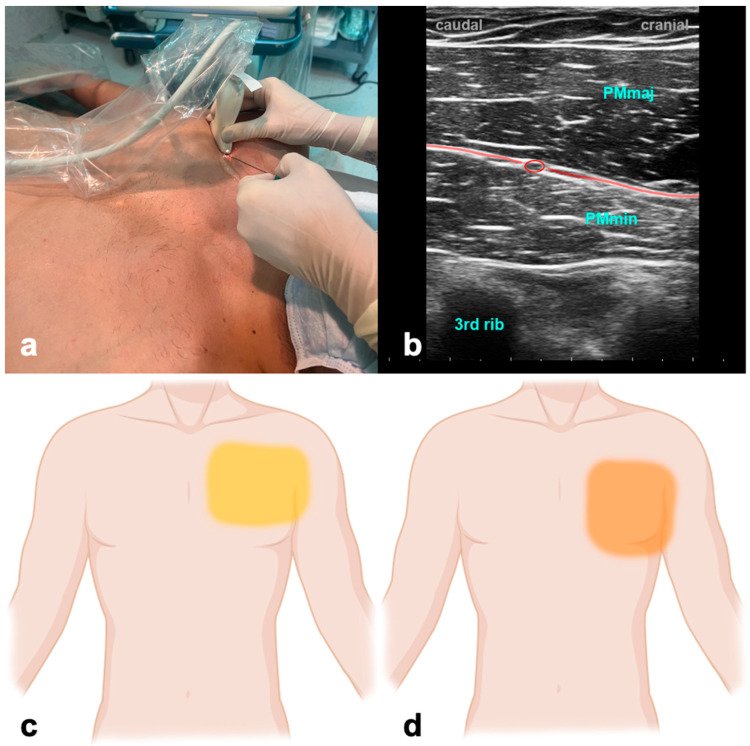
Pectoralis nerve block. (a) Transducer positioning. (b) Ultrasound anatomy of PECS I: PMmaj—pectoralis major muscle; PMmin—pectoralis minor muscle; red circle—pectoral branch of thoracoacromial artery; red line target space for PECS I block. (c) Spread of PECS I. (d) Spread of PECS II.
